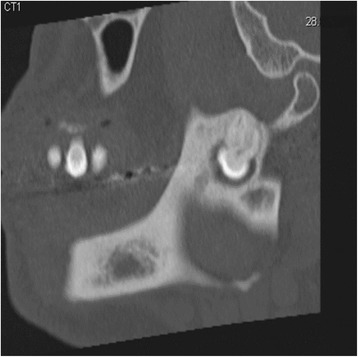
Sagital cone beam scan showing the impacted tooth with cranial-dorsal directed roots and bone apposition in the *right* sigmoid notch
